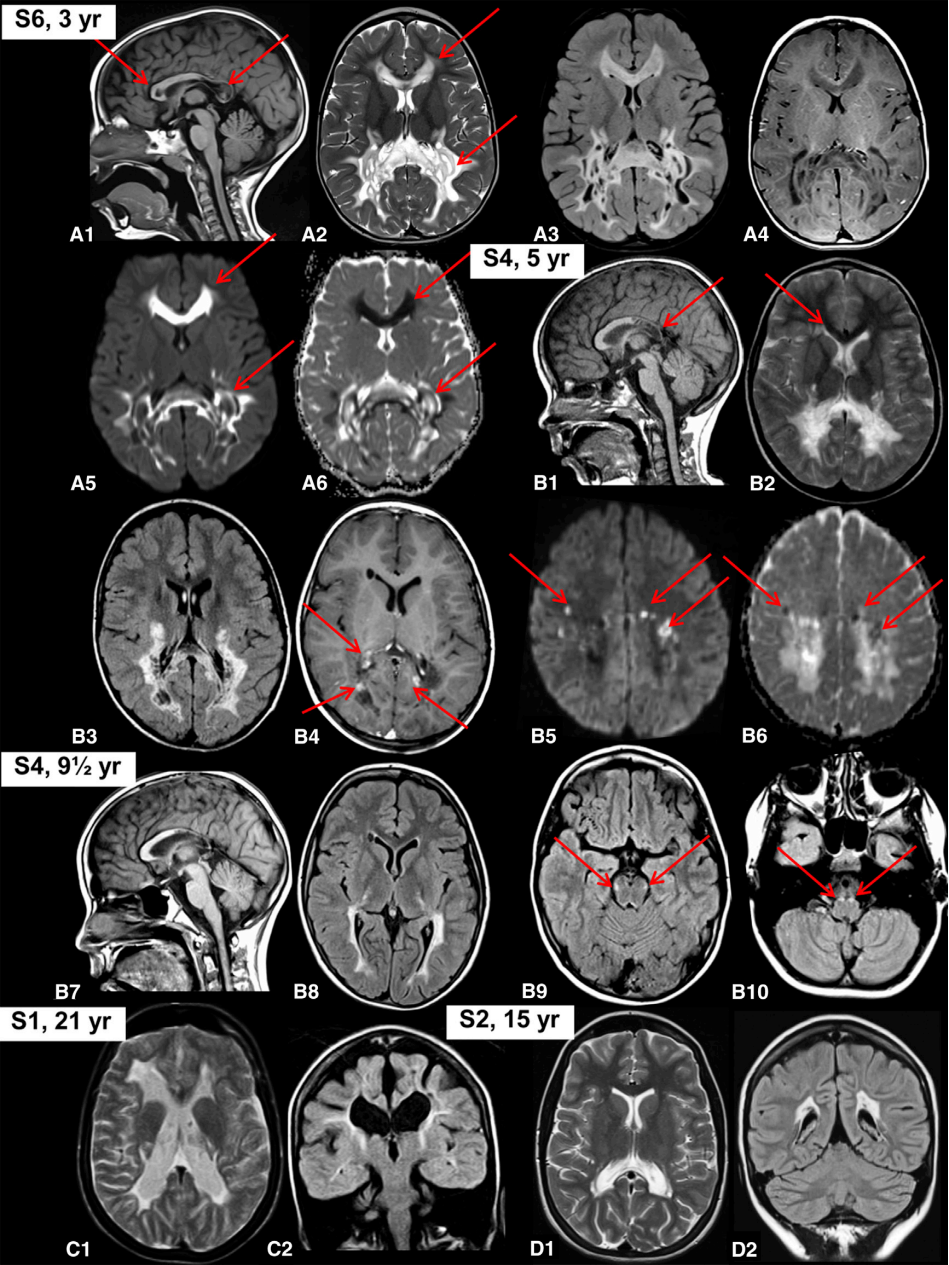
MRI Findings (A) MRI abnormalities observed in individual S6 in the acute stage at the age of 3 years. The sagittal image shows signal abnormalities in the posterior part of the corpus callosum and a single lesion at the genu (red arrows in A1). Axial T2-weighted (A2, red arrows), FLAIR (A3), and T1-weighted (A4) images show signal abnormalities predominantly involving the posterior part of the cerebral white matter and corpus callosum with numerous small and larger, well-delineated cysts. The diffusion-weighted images show that the noncavitated abnormalities have a high signal, suggesting diffusion restriction (red arrows in A5), as confirmed by the low signal on the apparent diffusion coefficient (ADC) maps (red arrows in A6). (B) MRI abnormalities observed in individual S4 in the subacute stage at the age of 5 years. The sagittal image shows the involvement of the posterior part of the corpus callosum (red arrow in B1). Axial T2-weighted (B2), FLAIR (B3), and T1-weighted (B4) images show signal abnormalities predominantly involving the posterior part of the cerebral white matter and corpus callosum with numerous small, well-delineated cysts. Additional minor abnormalities are seen next to the anterior horn of the lateral ventricle on the right (red arrows in B2 and B4). After contrast, enhancement of multiple foci is seen (red arrows in B4). The diffusion-weighted images show multiple small foci of high signal, suggesting diffusion restriction (red arrows in B5), as confirmed by the low signal on the ADC maps (red arrows in B6). Follow-up MRI of the same subject (B7–B10) shows striking improvement (B7 and B8). Involvement of long tracts within the brain stem is now visible (red arrows in B9 and B10). (C) Late follow-up MRI of individual S1 at age 21 shows atrophy and gliosis of what is remaining of the cerebral white matter (C1) with some small cysts in the abnormal white matter (C2). (D) MRI of individual S2 shows only minor posterior cerebral white matter abnormalities at age 15 (D1) with tiny cysts (D2).
